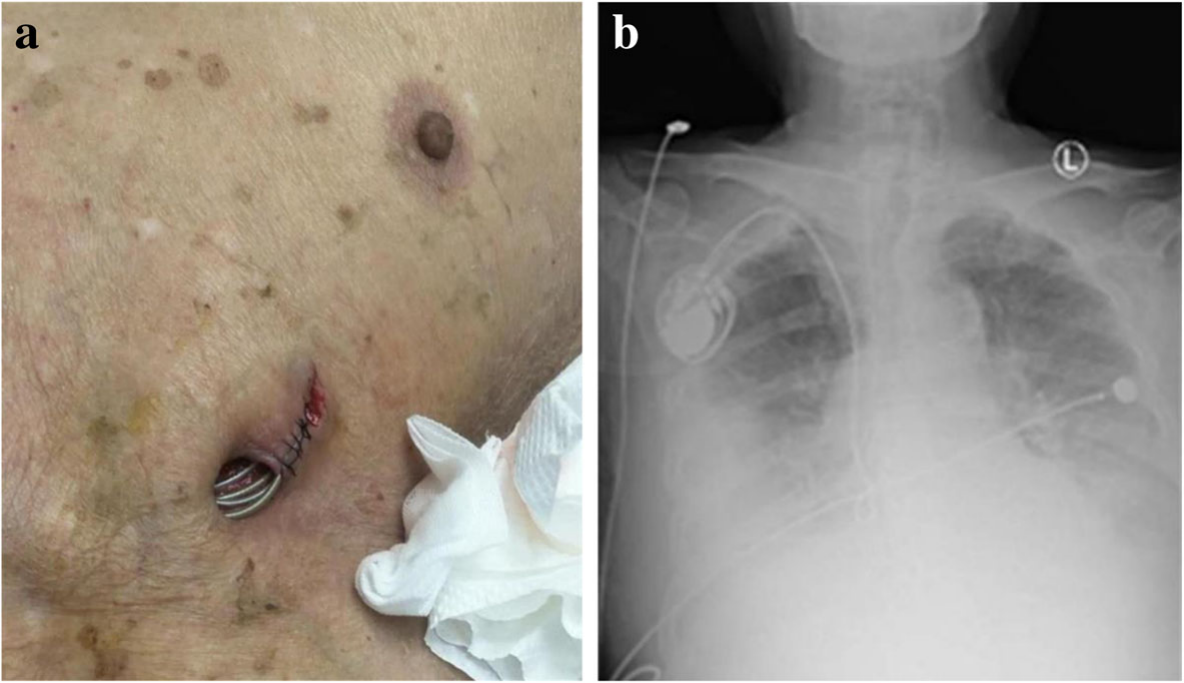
a Appearance of the wound after irrigation (second week in the hospital). b Preoperative chest X-ray showed the generator was in the infraclavicular region
